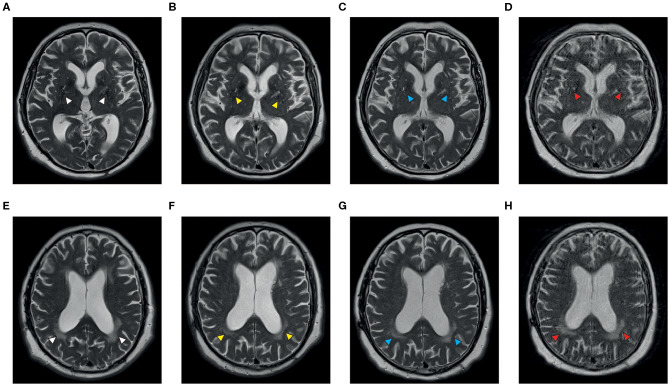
Axial T2-weighted MR images of a patient with NPSLE before (A,B,E,F) and during treatment with belimumab (C,D,G,H). Vascular white matter lesions can be demonstrated in the region of the basal ganglia (A) and the posterior periventricular matter (E) at the onset of neuropsychological symptoms (white arrowheads); there is a progress of the central lesions and formation of anterior white matter involvement within 15 months while the posterior lesions appeared less intense (B,F, yellow arrowheads). The panels on the right demonstrate stable disease after four (C,G, blue arrowheads) and 22 months (D,H, red arrowheads) of treatment with belimumab, respectively. Images courtesy of Prof. Dr. C. Riedel, Department of Neuroradiology, University Medical Center Göttingen.
